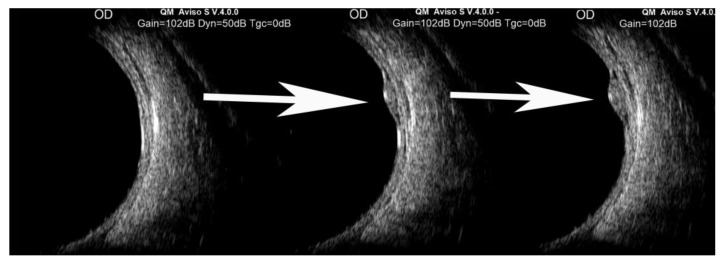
A series of three sequential B-scan ultrasound images capturing intumescence of a vortex vein ampulla varix. During clinical exam, this was observed dynamically as light digital pressure was released from the pre-equatorial quadrant where the lesion was located.
